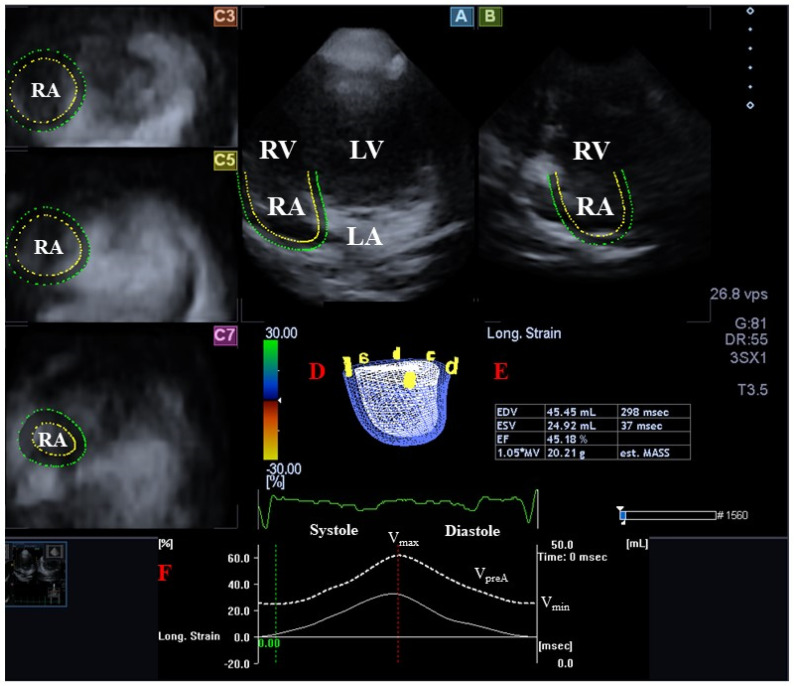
Assessment of the right atrium (RA) via three-dimensional (3D) speckle-tracking echocardiography using apical longitudinal four-chamber (A) and two-chamber (B) views, and short-axis views at basal (C3), midatrial (C5), and superior RA level (C7). Three-dimensional model of the RA (D), calculated RA volumetric data (E), and time— RA global volume change (white dotted line) during the cardiac cycle are also presented (F) together with maximum (V_max_), preatrial contraction (V_preA_), and minimum (V_min_) RA volumes. Abbreviations: LA, left atrium; LV, left ventricle; RA, right atrium; RV, right ventricle; EDV, end-diastolic volume; ESV, end-systolic volume; EF, ejection fraction; V_max_, end-systolic maximum RA volume; V_preA_, early diastolic pre-atrial contraction RA volume; V_min_, end-diastolic minimum RA volume.
